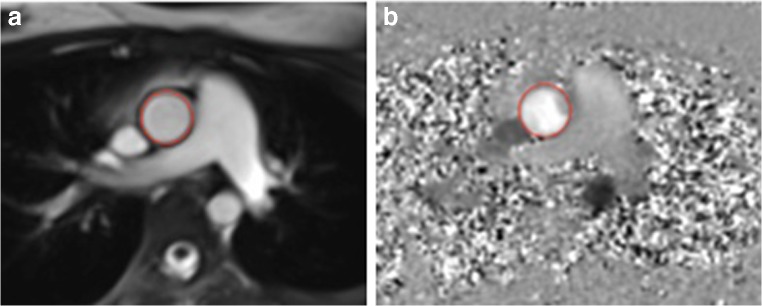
Phase contrast MR images acquired at the level of the ascending aorta of a 15 year old boy. a Magnitude image, (b) phase image. The region of interest (*circles*) traces the outline of the cross-sectional aorta area throughout all phases of the cardiac cycle to obtain accurate cardiac output for calculating systemic vascular resistance and total arterial compliance
